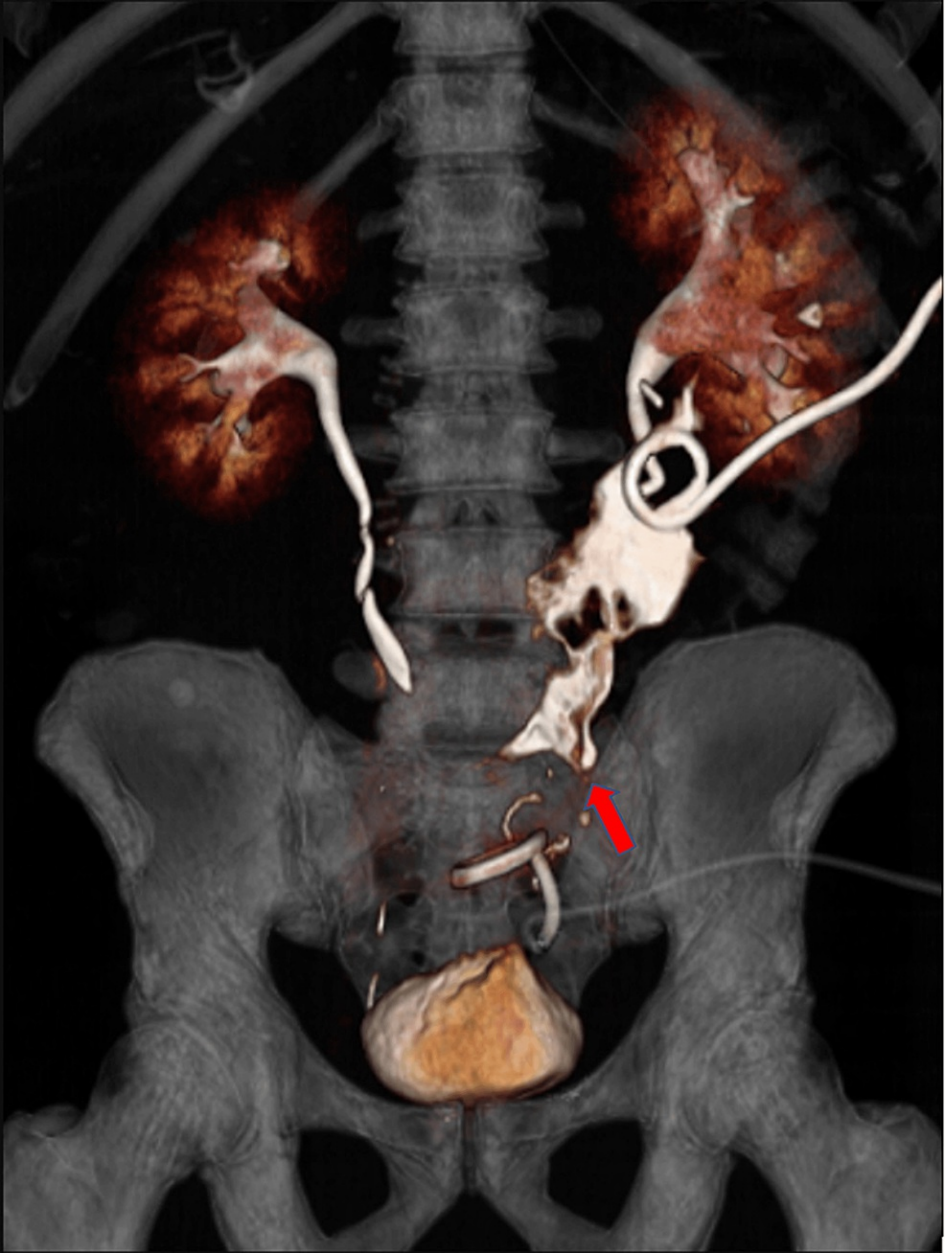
Computerized tomography urogram A virtual reality reconstructed CT urogram shows the escape of contrast material (red arrow) into the area of the previously drained fluid collection.
